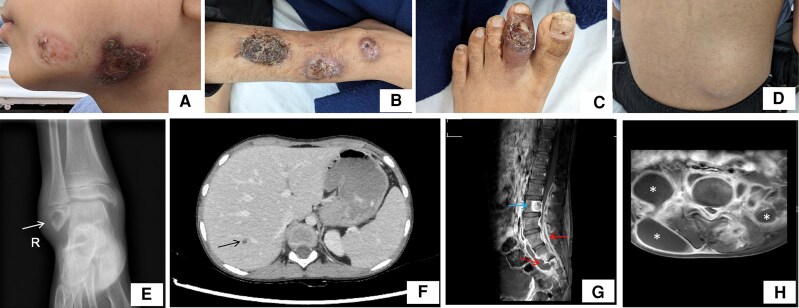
A*, Shows diffuse swelling of the left mandibular region with overlying two erythematous, well-defined, crusted plaques discharging seropurulent exudate. *B*, Three erythematous, “boggy” plaques with crusting and purulent discharge on the right lower leg. *C*, Fusiform swelling with ulceration and crusting of the left second toe. *D*, Shows a localized, nontender, nonerythematous swelling measuring 8 × 7 cm on the right para-spinal area. *E*, Radiograph showing a lytic lesion in the right fibula. *F*, CECT abdomen revealing a hypodense (granulomatous) lesion in the liver. *G*, T1-weighted fat-suppressed postcontrast image in sagittal plane shows enhancement in L4 (blue arrow), S2, and S3 vertebral bodies. There are associated prevertebral and intradural epidural abscesses extending from L3 to S4 (red arrows). *H*, Axial plane MRI demonstrates multiple abscesses in the bilateral paravertebral region involving psoas muscles and erector spinae muscles, reaching up to the skin (*). Inflammation also extends into the epidural space.
